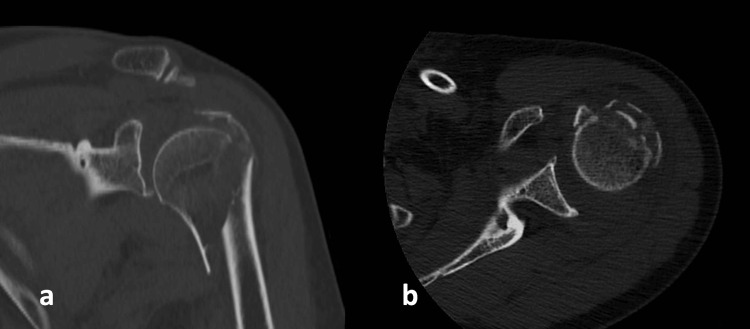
CT scan with coronal (a) and axial (b) views showing multi-fragmented proximal meta-epiphyseal fracture of the humerus (Case 1)
